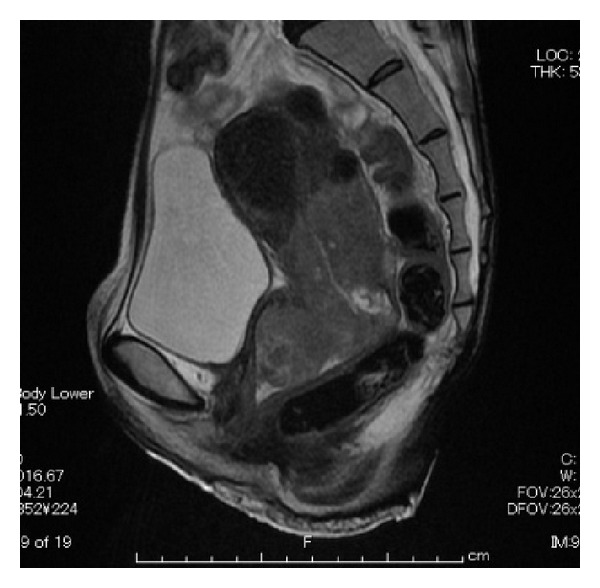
MRI (T2-weighted) reveals a large mass infiltrating to the uterine corpus and vagina.
